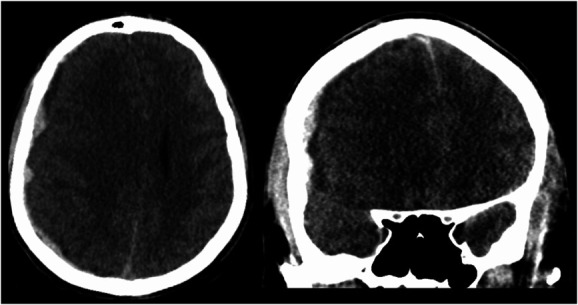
Computed tomography scan of a right-sided acute-on-chronic subdural hematoma in an axial and coronal plane.
